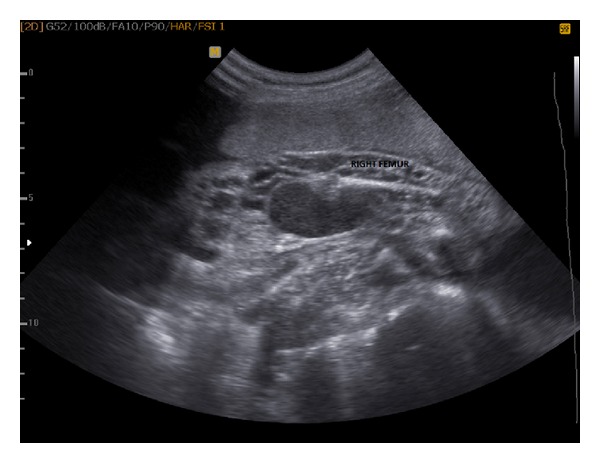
Longitudinal ultrasound image of the right thigh at 25 weeks and 4 days of gestation. Multiple cystic areas over the entire segment shown. This pattern continued below the knee, but the foot was not involved.
